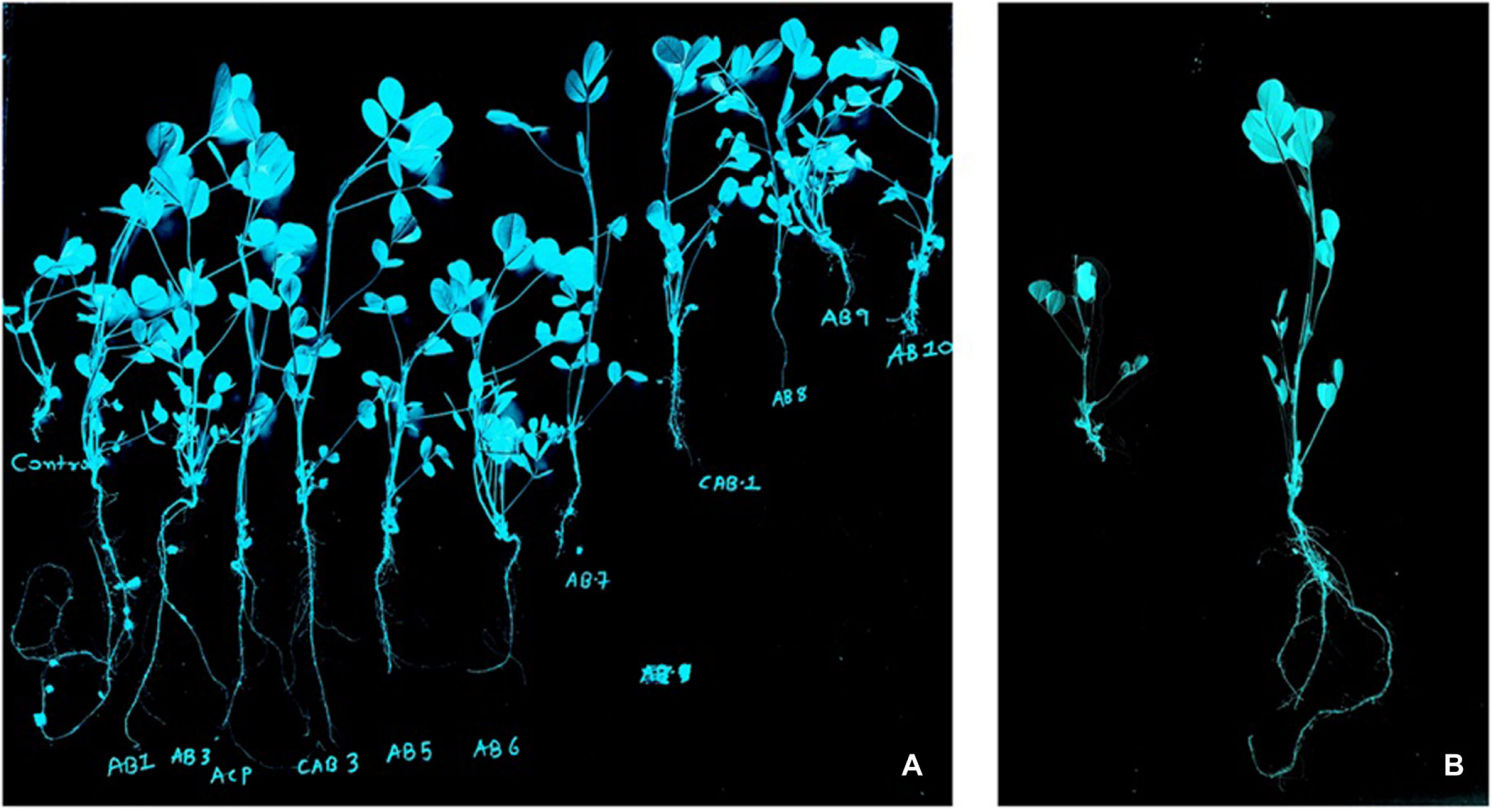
Radiographic images of pot study of *Arachis hypogaea L*. Image (A) showed comparative results of all isolates against control, and image (B) shows *Bacillus rugosus* AB1 against control.
